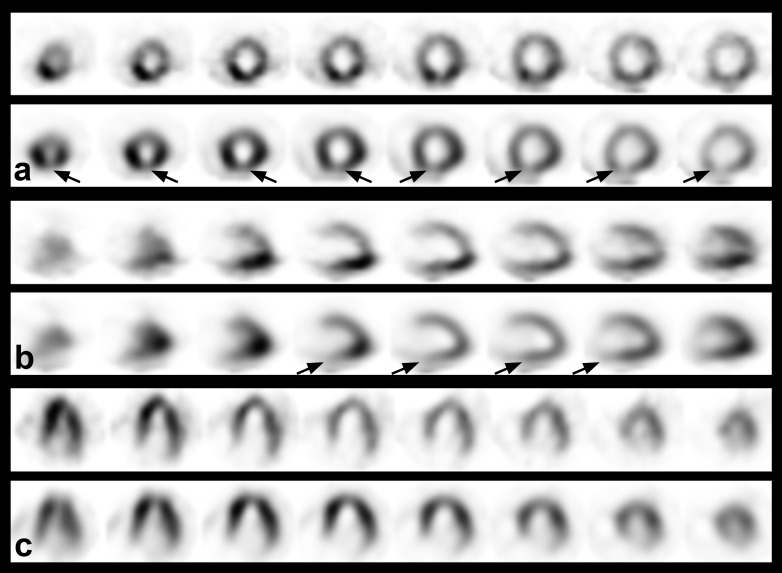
Stress and Rest 99mTc-MIBI single-photon emission computed tomography images aligned in standard short axis (a), vertical long axis (b), and horizontal long axis (c) format revealing an area with reduced 99mTc-MIBI uptake at rest imaging in the lower wall segment (reverse perfusion pattern).
